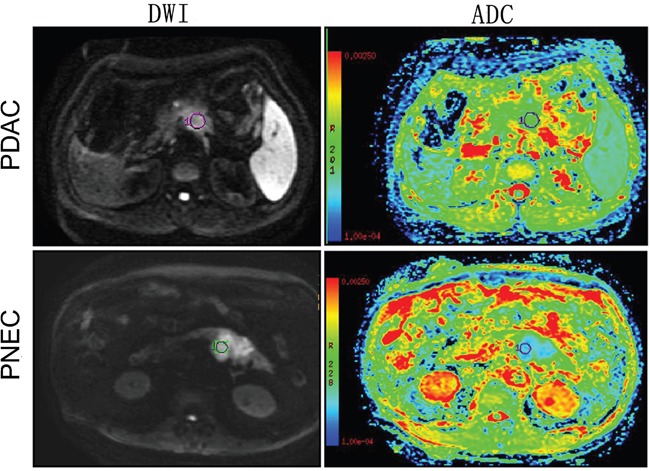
Diffusion-weighted images and ADC maps in pancreatic ductal adenocarcinoma (PDAC) and pancreatic neuroendocrine carcinoma (PNEC) PNEC showed higher DWI signal and lower ADC value than PDAC.
